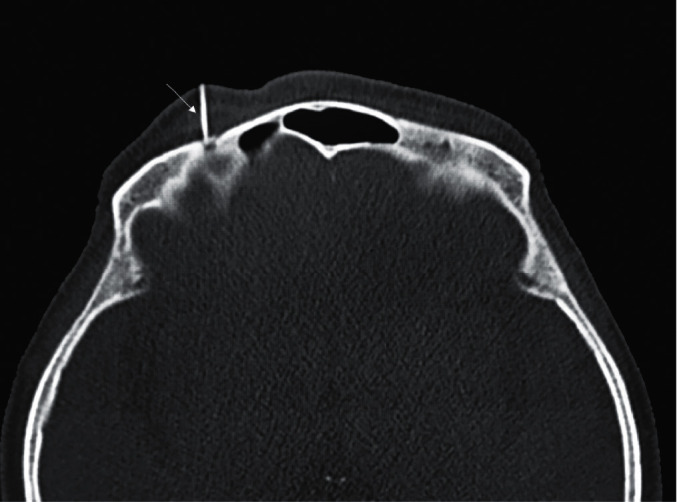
Computed tomography scan showing that the tip of the radiofrequency needle was located in the supraorbital foramen. The white line entering the skull is the radiofrequency needle (with white arrow pointing).
